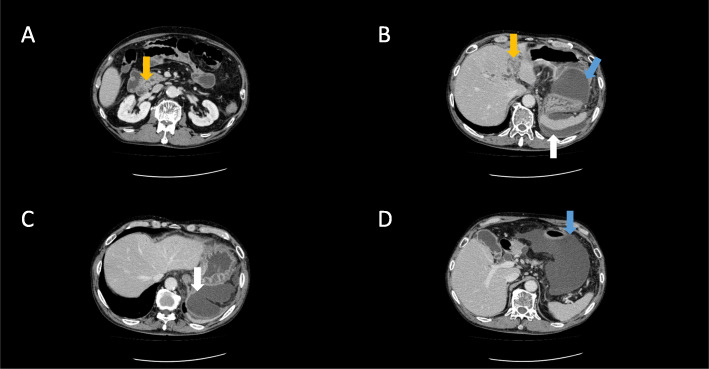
Contrast-enhanced computed tomography findings. Scans showed the following: (a) a dilated common bile duct with choledocholithiasis (orange arrow); (b) intrahepatic biliary dilatation (orange arrow), an intra-abdominal abscess surrounded by smoothly thickened and enhanced peritoneum (white arrow) and a gastric submucosal abscess (blue arrow); (c) an intra-abdominal abscess surrounded by smoothly thickened and enhanced peritoneum (white arrow); and (d) a gastric submucosal abscess (blue arrow)
